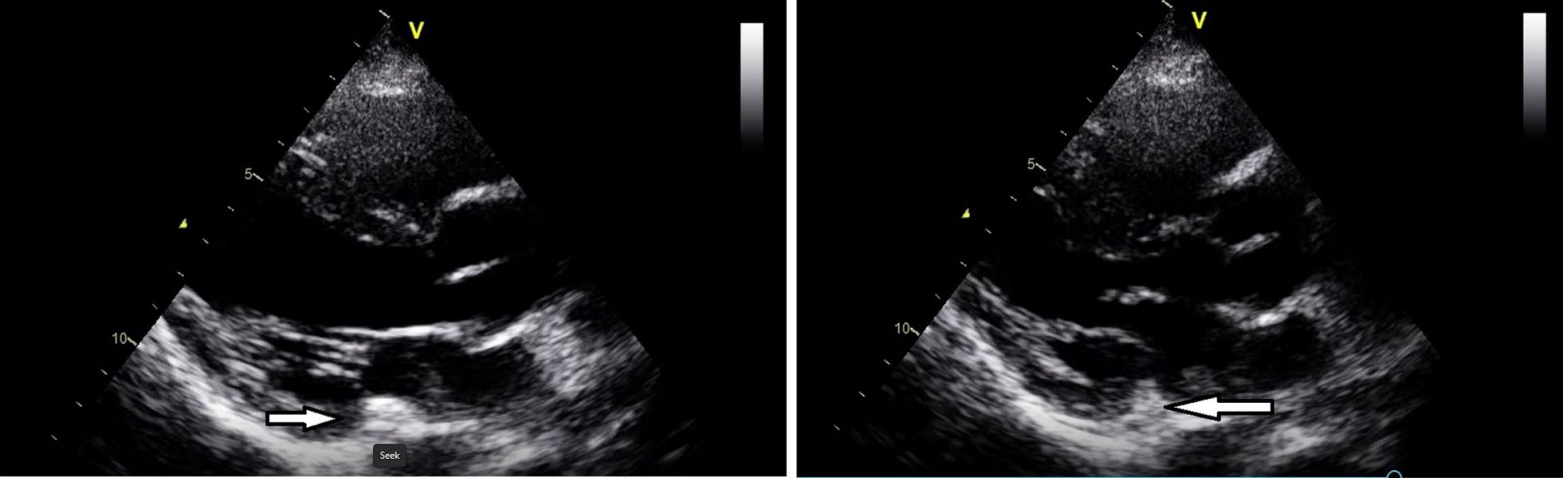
Echocardiography showing mobile, finger-like echogenic density in left atrium shown with white arrow
